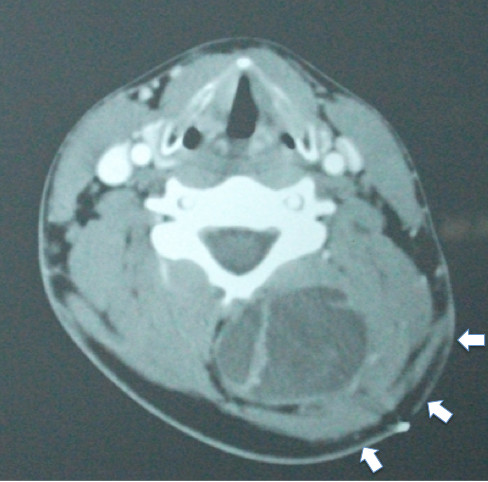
Axial CT scan of the cervical spine. 3 mm transaxial CT scan with nonionic intravenous contrast. The non-enhancing cystic mass involves the paraspinal musculature and is adjacent to the posterior elements particularly the spinous process and laminae of C4. Note the prominent distortion of the soft tissues overlying the tumor (white arrows) when compared to the unaffected side.
